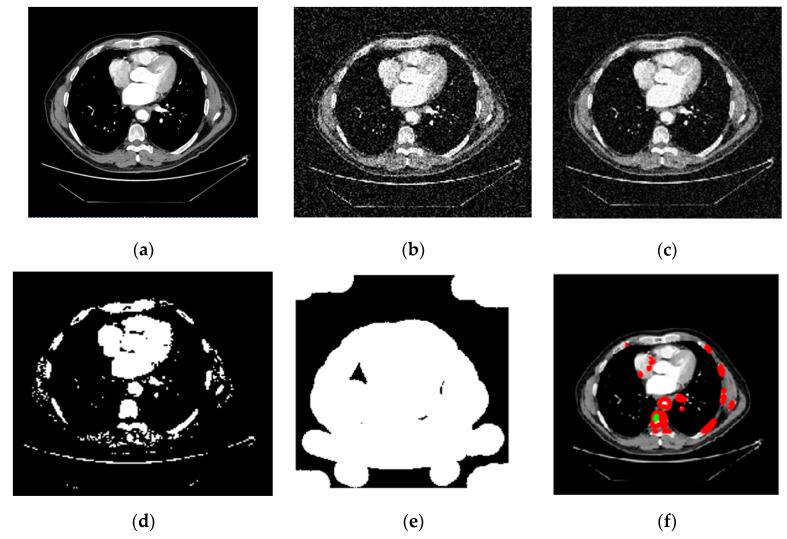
CT Image classification procedure. (a) Original Image. (b) Noisy Image. (c) Adaptive Filtering PSNR. (d) Dilate image. (e) Watershed Transform. (f) Lung cancer type: malignant.
